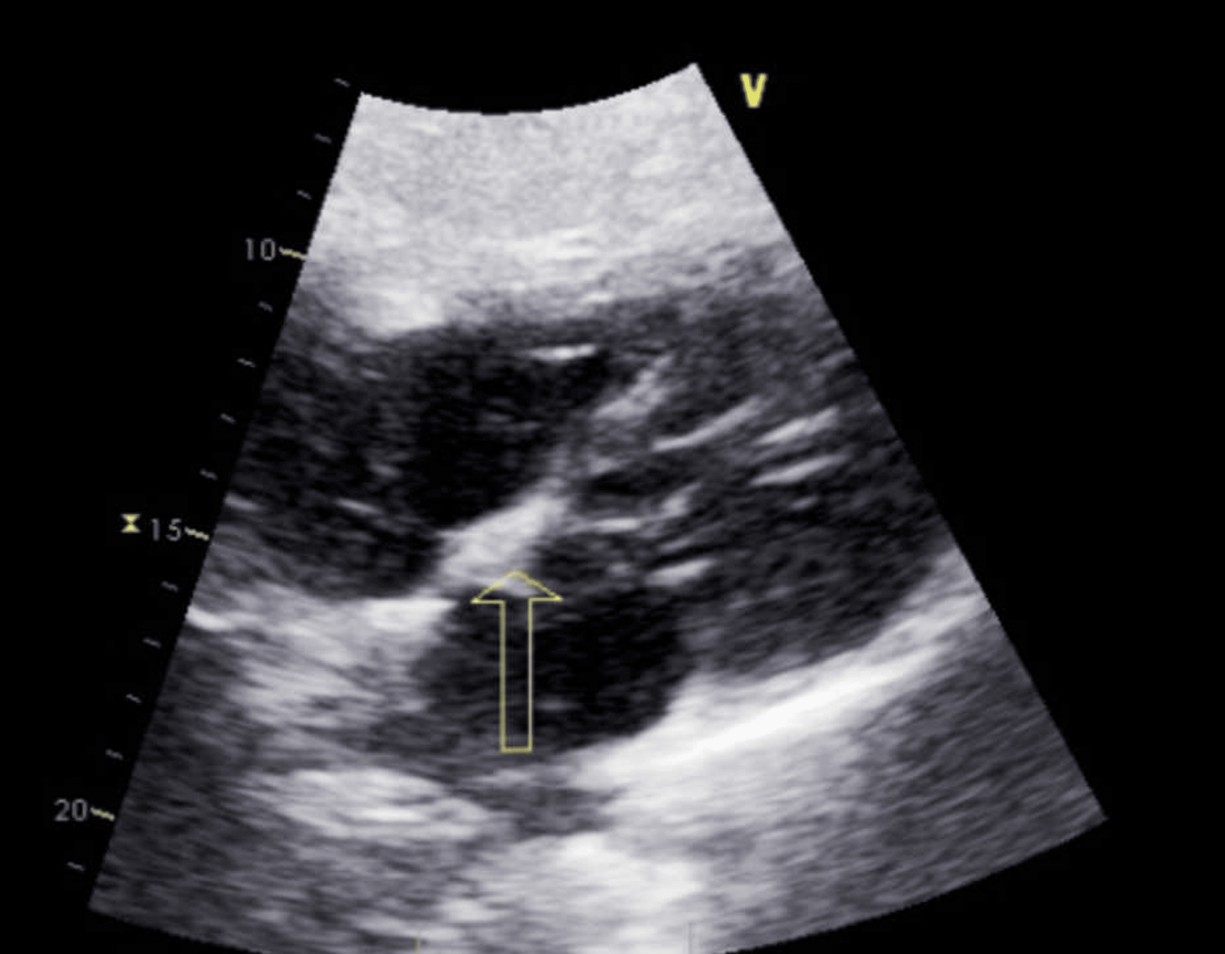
Transthoracic echocardiogram showing a mobile 1.7 cm x 0.4 cm echodensity attached to the tricuspid valve, suggestive of vegetation (yellow arrow).
